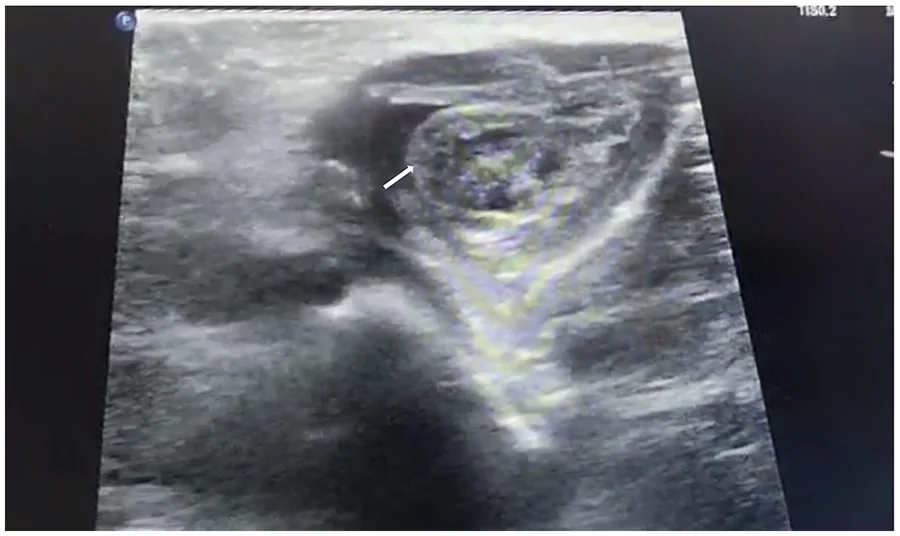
(A) Ultrasound image of the urinary bladder demonstrating an intraluminal, well-defined heterogeneous mass (arrow), with surrounding urine.
